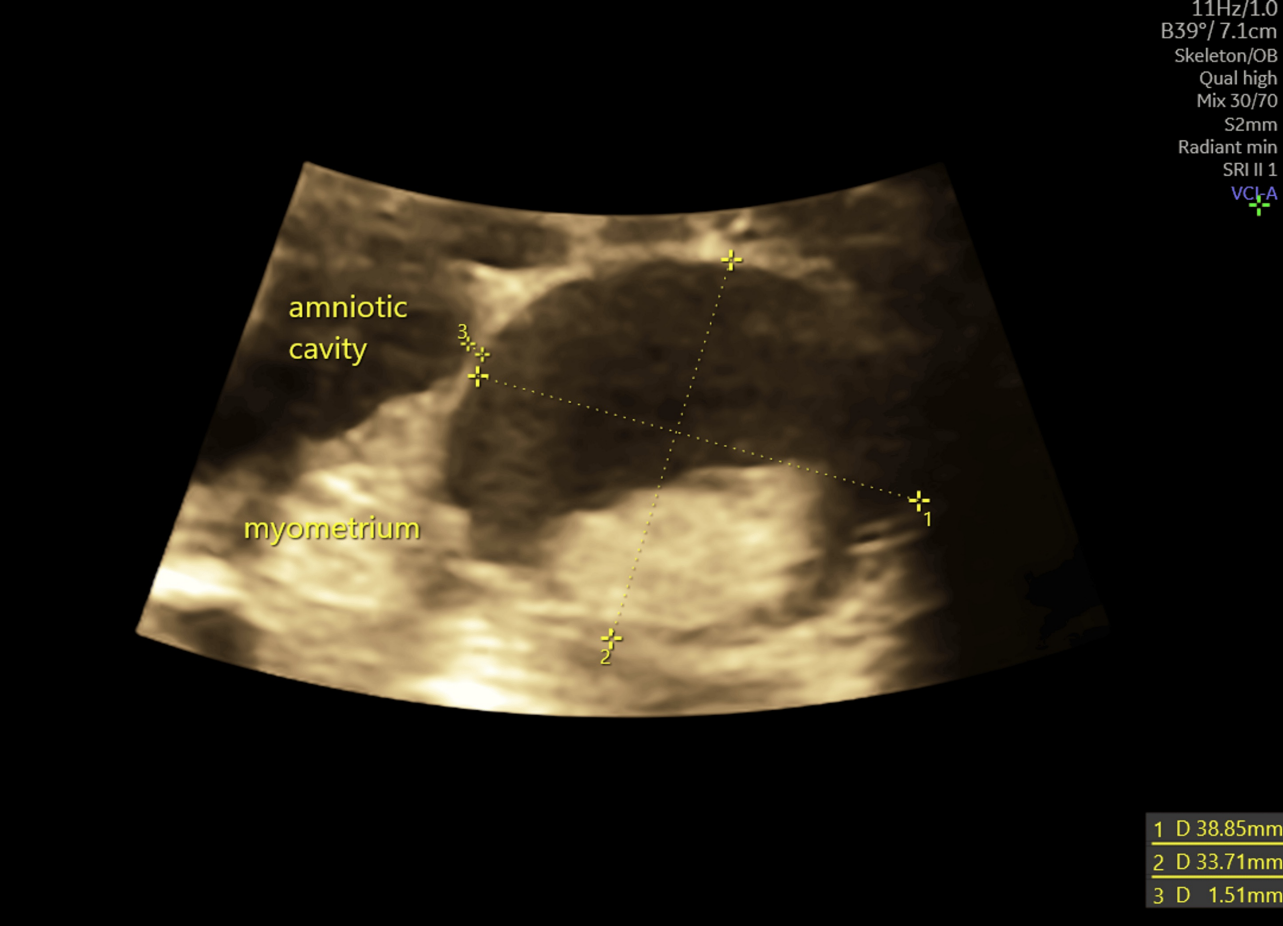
Transabdominal ultrasound at 22 weeks + five days (second trimester), transverse uterine view demonstrating an ACUM with hyperechoic content, measurements in two orthogonal planes, and the distance from the amniotic cavity. ACUM: accessory cavitated uterine malformation
